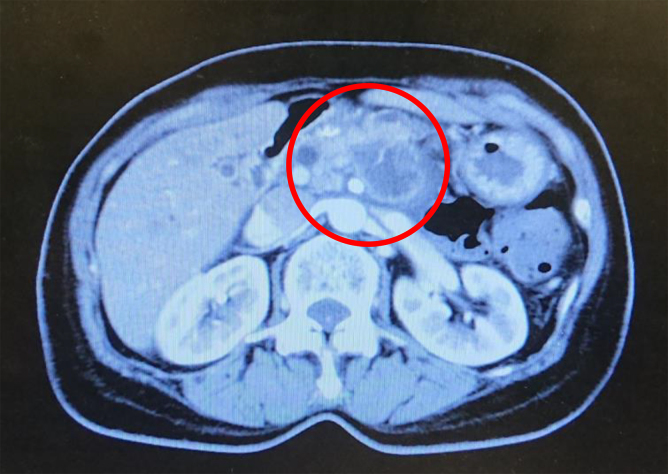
Abdominal computed tomography image of the first patient. A tumor of ∼36 mm in the main body of the pancreas (red circle), which appeared to have invaded the splenic artery, celiac artery, and portal vein was displayed.
